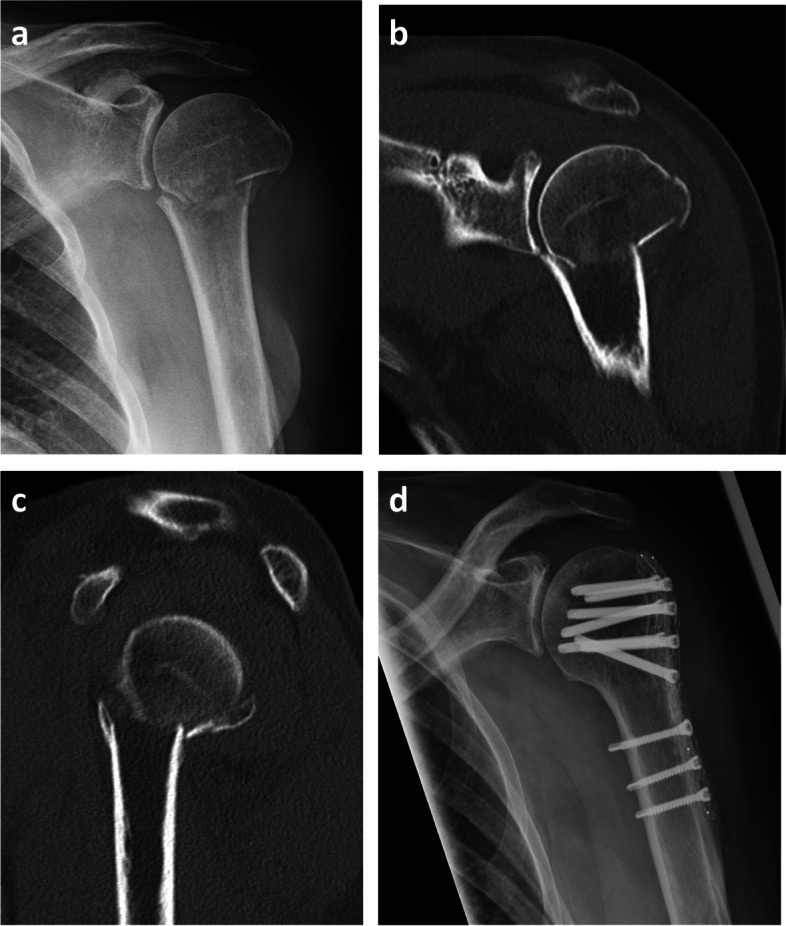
Radiological imaging of a 3-part proximal humerus fracture 69-year-old female patient with 3-part proximal humerus fracture treated with CFR-PEEK plate osteosynthesis; preoperative imaging: a) radiograph in ap view, b) CT scan in coronal plane, c) CT scan in sagittal plane; d) postoperative radiograph in lateral view. (CFR-PEEK = carbon fibre-reinforced polyetheretherketone, CT = computed tomography)
